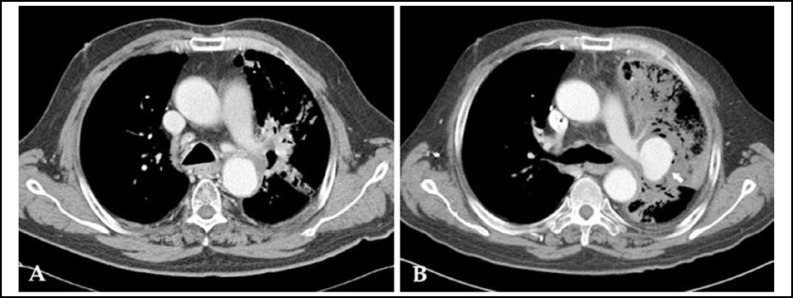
Computed tomography findings of the pulmonary artery before and after the occurrence of the pulmonary pseudoaneurysm
